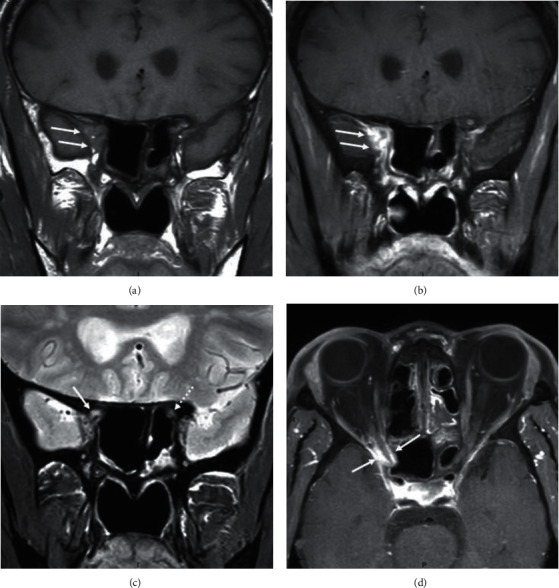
Orbital magnetic resonance imaging. (a) Coronal unenhanced T1-weighted view showing filling of the right sphenoid fissure by inflammatory material (double arrow). (b) Coronal contrast-enhanced T1-weighted view with fat suppression option in similar slice location as (a) showing intense enhancement of the inflammatory material (double arrow). (c) Coronal T2-weighted view showing abnormal hyper signal intensity of the intracanalar segment of the right optic nerve (arrow) when compared with normal contralateral side (dotted arrow). (d) Coronal contrast-enhanced T1-weighted view with fat suppression option showing a “tramtrack”-like encasement of the intracanalar segment of the right optic nerve (between arrows).
